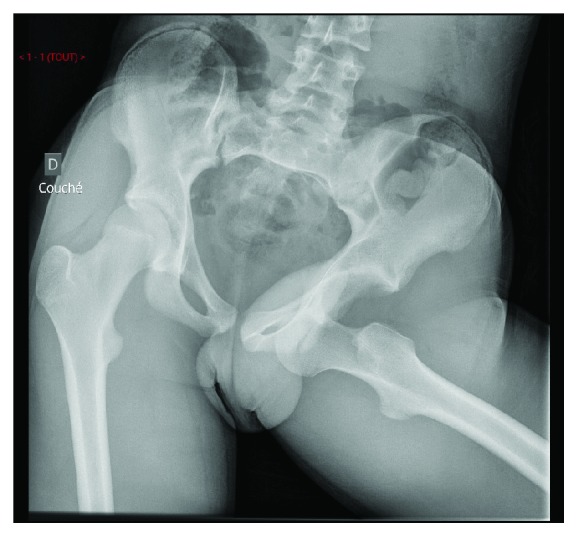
Emergency pelvic X-ray AP view.
